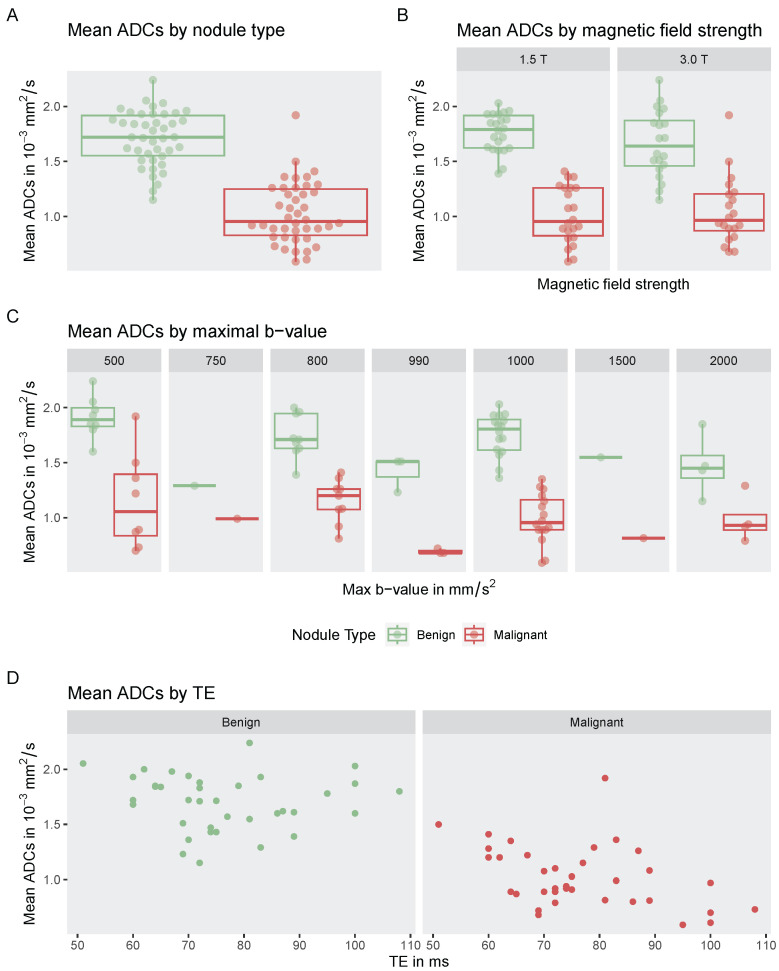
Mean ADC values (in $10^{-3}\;{\mathrm{mm}}^{2}/s$) reported by the included studies for (A) benign and malignant nodules, (B) stratified by magnetic field strength (in Tesla) of the MRI scanner used, and (C) stratified by the maximal b-value (in s/mm^2^) used in the respective studies. (D) Mean ADC values plotted against echo time (TE in ms), stratified by nodule type.
